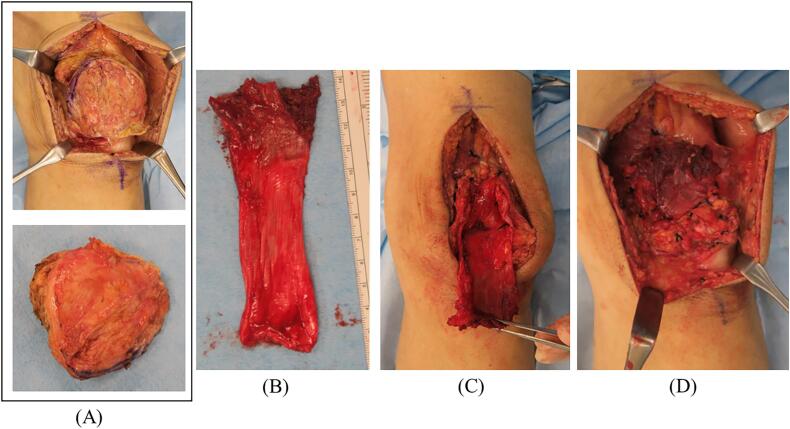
Preoperative images of the malignant scapular tumor in the right shoulder. On magnetic resonance imaging, the frontal view of the right shoulder (A) showed malignant tumor invasion of the entire scapula and glenoid fossa but not the acromioclavicular joint. The axial view of the right shoulder (B) showed malignant tumor invasion of the deltoid muscle (abductor muscle). Three-dimensional computed tomographic angiography (C) showed several feeding vessels extending from the axillary artery to the malignant tumor.
